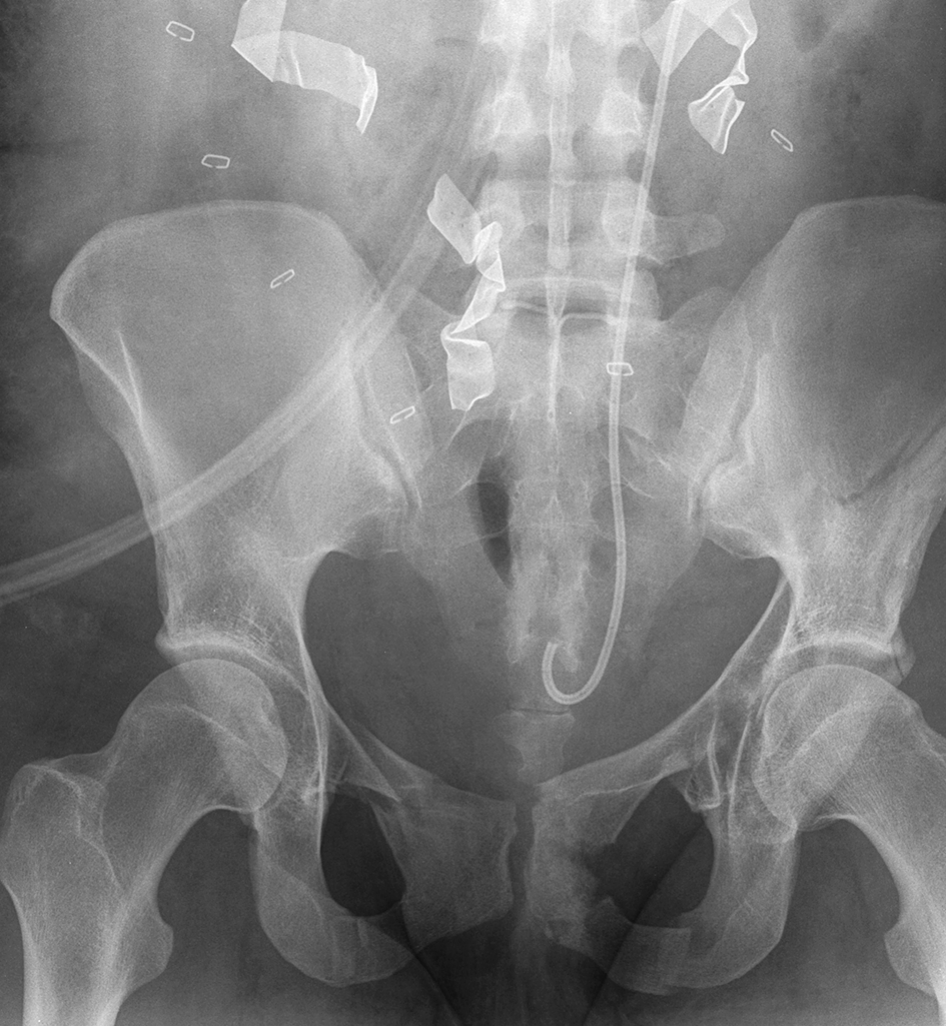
Plain pelvic x-ray (AP view) of the patient on admission showing extensive pelvic fractures
